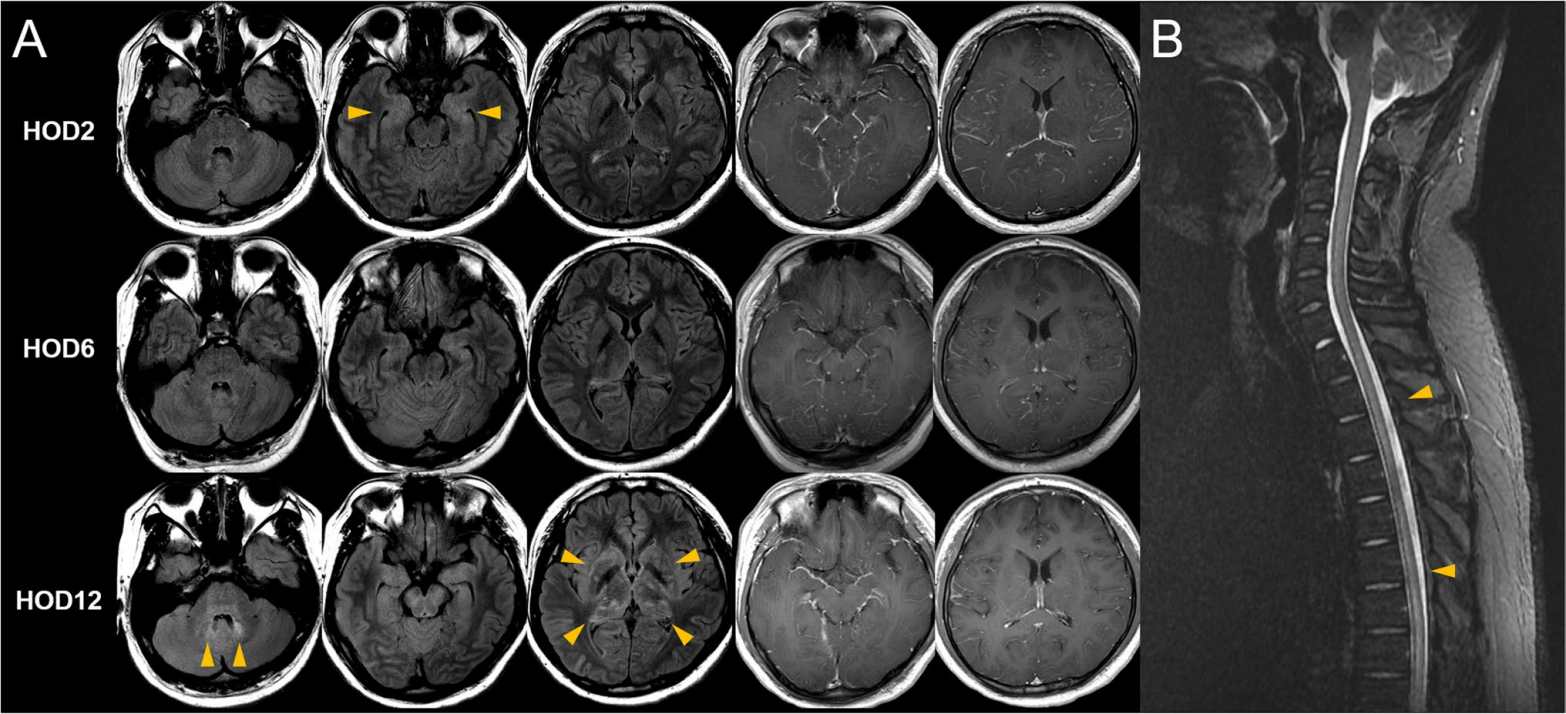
The brain and spinal MRI findings.
